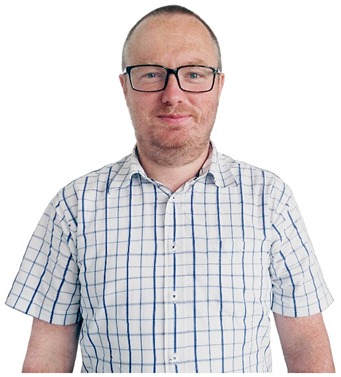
Dr. Jan Van den Stock is a clinical psychologist and post-doctoral FWO-fellow at KU Leuven, Belgium. His professional track record consists of a combination of research and clinical activities. His research focuses on the behavioral and neural correlates of socio-emotional processing in neurodegenerative disorders. Dr. Van den Stock combined his PhD-track with neuropsychological work in ambulant and residential neurology and psychiatry settings. In 2010, he obtained his PhD (Bodies and background: Contextual influences on perception of emotional body expressions). For his first postdoc term (FWO), he moved back from the Netherlands (Tilburg University) to Leuven and co-founded the laboratory headed by Prof. Dr. Mathieu Vandenbulcke to build on the work of his PhD. He investigated the behavioral and neural underpinnings of emotion and body perception in normal and clinical populations using psychophysical and functional and structural imaging [(f)MRI] techniques. Currently, Dr. Van den Stock is in the fifth year of his post-doc track. He has (co)authored 37 peer-reviewed articles and they are frequently cited (H-index = 15, according to Web of Science) and include recommendation by Faculty of 1000 (http://f1000.com/prime/13339967). Furthermore, he has organized research symposia with international speakers. He has taught five psychology and psychiatry courses at EHSAL Brussels (graduate school) and three neuropsychology and research courses at Tilburg University. He supervised graduate students for either their master’s thesis or research section of their clinical internship. He is co-promotor of two PhD-students. Regarding funding, he obtained grants from Tilburg University, FWO (post-doc fellowships and research grant), KU Leuven and Foundation for Alzheimer Research (SAO-FRA). Furthermore, he has been solicited as a reviewer for leading journals like *PNAS*, *Biological Psychiatry*, *Current Biology*, *eLife*, *NeuroImage*… This year, he received a special reviewer recommendation from the editorial board of PLoS ONE.
